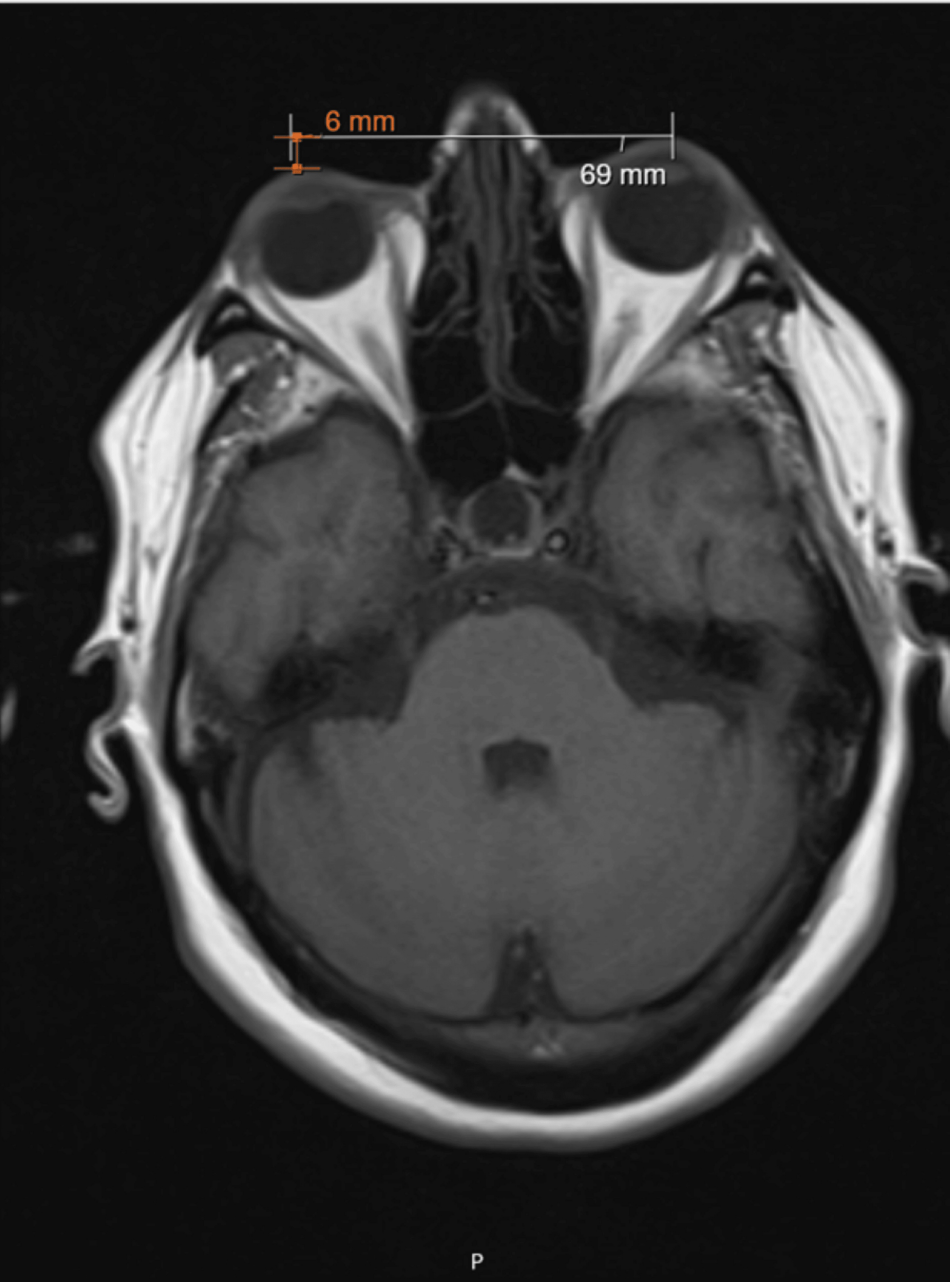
Orbital MRI (T1-weighted sequence) This image demonstrates a 6 mm asymmetry with anterior displacement of the left globe, consistent with unilateral proptosis. MRI, magnetic resonance imaging
